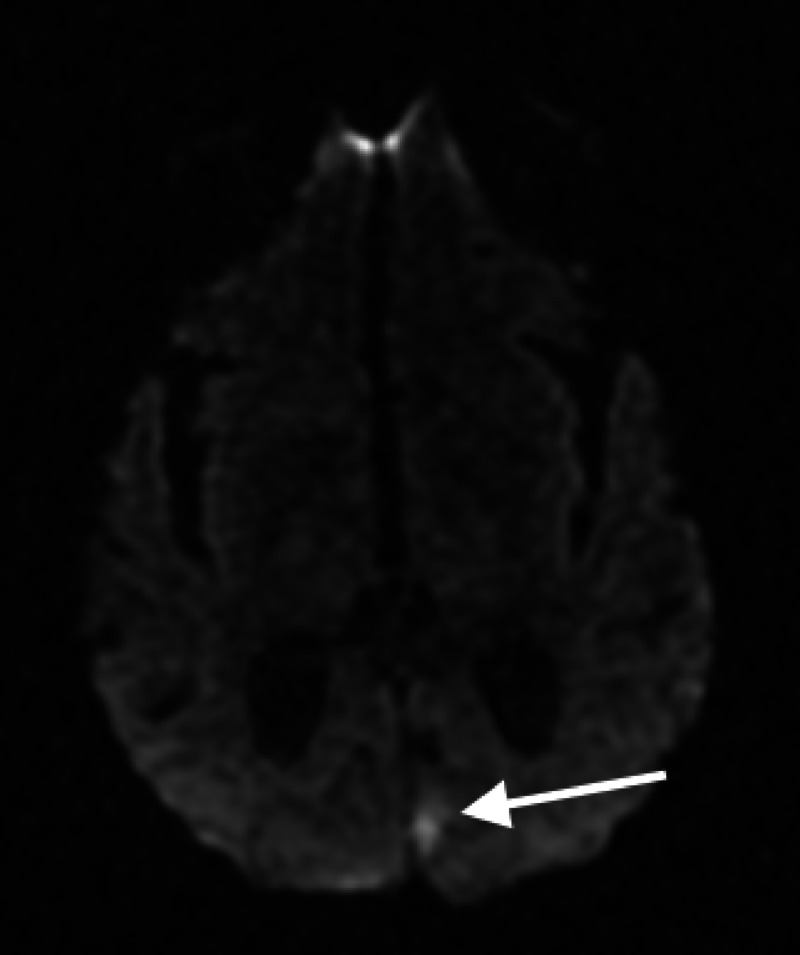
MRI: (DWI) demonstrating ischemic infarct of striate cortex, V1, and associated visual association cortex, V2 (white arrow) MRI: Magnetic Resonance Image; DWI: Diffusion-Weighted Imaging
